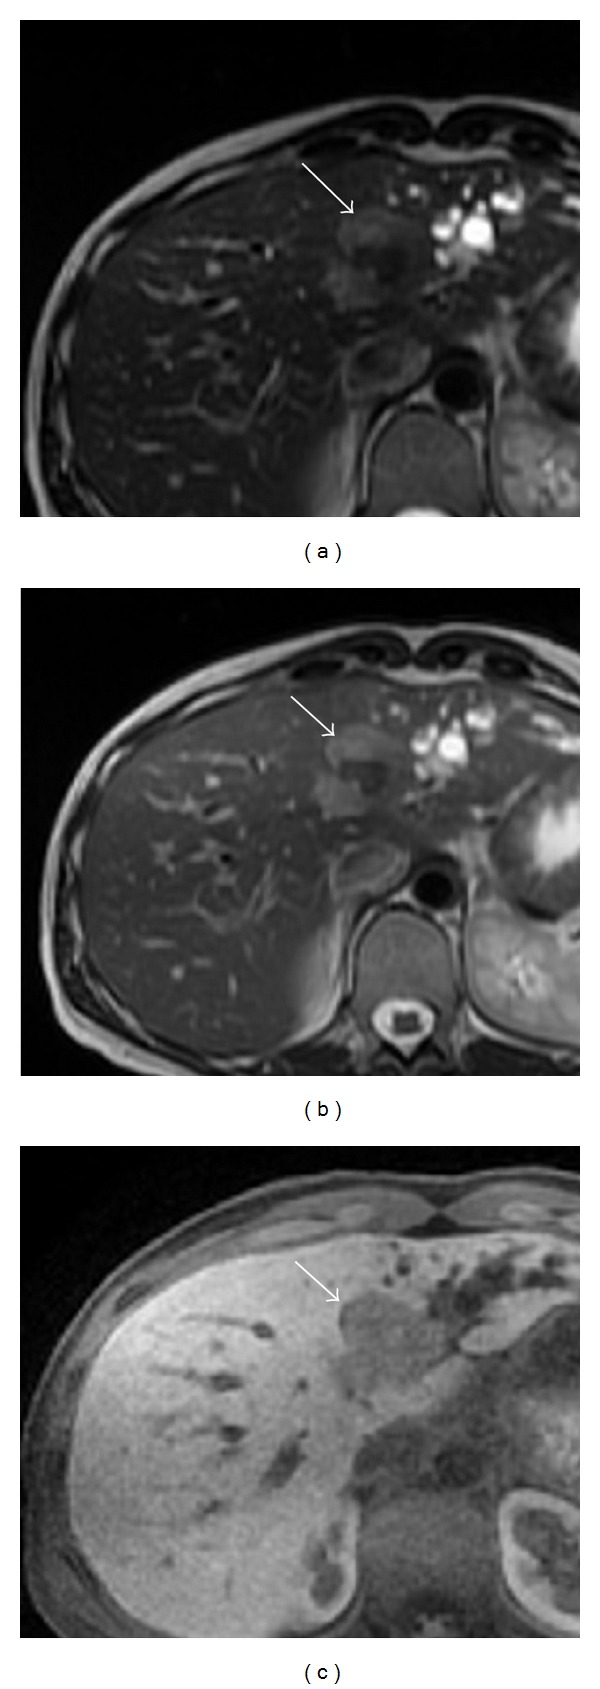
MRI of a lobulated lesion in segments 2 and 4 (white arrow). (a) T2 weighted first echo. (b) T2 weighted second echo. (c) Delayed liver acquisition with volume acquisition (LAVA).
